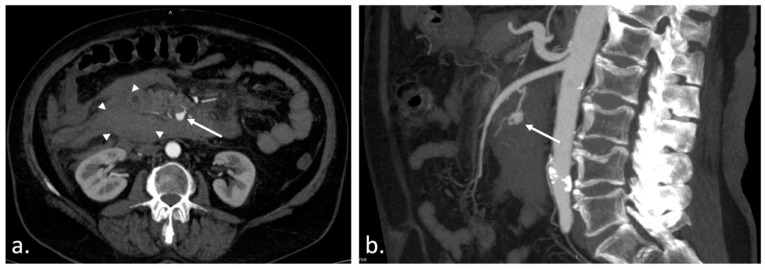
Aneurysmal dilatation of visceral arteries occurs in less than 1% of arterial aneurysm cases [1,2], primarily affecting the splenic artery, hepatic artery, and superior mesenteric artery; in only 1–2% of visceral aneurysms, it is found in the pancreaticoduodenal or gastroduodenal artery [1], with a high mortality rate in untreated cases [2,3,4]. Arterial aneurysmal dilatation develops as a result of damage to the collagen structure at the arterial wall caused by atherosclerosis or hypertension, and is found with the highest incidence at the level of the abdominal aorta [5,6,7,8,9]. In contrast, arterial pseudoaneurysms are connected with trauma, surgical treatments, or the presence of malignancies, but in the case of the pancreaticoduodenal artery, the main reason is the association with chronic pancreatitis [9,10,11,12,13,14,15,16]. Although it is a rare pathology, it is lethal in the absence of intervention. Endovascular treatment is currently the primary approach in the event of these pathologies, with a high success rate and excellent patient progression [4,9,13,14,15,16,17,18,19,20,21,22,23]. A 67-year-old woman presented with lightheadedness, diaphoresis, and acute epigastric and right hypochondrium pain. Along with other antecedents, her past medical history includes stage 2 essential hypertension, chronic ischemic cardiomyopathy, and class 1 obesity. Furthermore, three weeks before her current admission, she experienced a SARS-CoV-2 infection. She has no history of alcohol abuse, smoking, or abdominal trauma. An abdominal contrast-enhanced CT scan showed an extensive hematoma (3 × 4 cm^2^ in size) located intra-abdominally, adjacent to the duodenojejunal area, with hyperdensity around the duodenum and inferior to the pancreas (30–59 HU). Moreover, the CT scan also revealed an enhancing lesion as a pseudoaneurysm of the inferior pancreaticoduodenal artery measuring 5 × 8 × 8 mm^3^ with active bleeding and an associated hematoma. Additionally, the pancreas showed multiple hyperdense hemorrhagic structures anteriorly and superiorly under the aspect of possible acute hemorrhagic-ulcerative pancreatitis. Following these investigations of the abdominal area, a decision was made to proceed with an endovascular intervention within the interventional radiology department. (a) Transverse CT angiogram image shows a 12 mm enhancing lesion (white arrow) and a large intraperitoneal hematoma (arrowheads); (b) Sagittal MIP image depicts the enhancing lesion as a pseudoaneurysm (white arrow) of the inferior pancreaticoduodenal artery, responsible for the bleeding.
